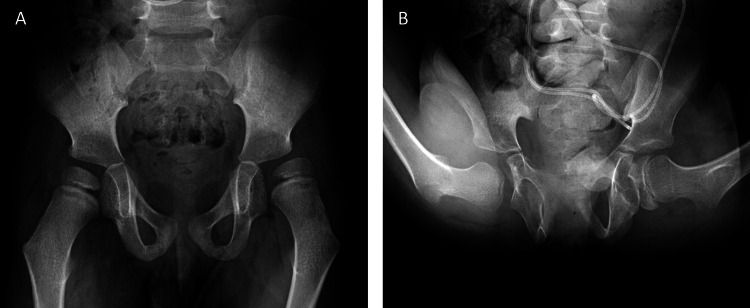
Case 2 X-rays, preoperative (A) and postoperative (B). Gross Motor Function Classification System level 5. On the preoperative pelvis X-ray, the left hip has a migration percentage (MP) of 55. At six years postoperative, the left hip has an MP of 25. The hips were held flexed for the X-ray to compensate for increased lumbar lordosis and to get a good anteroposterior view.
